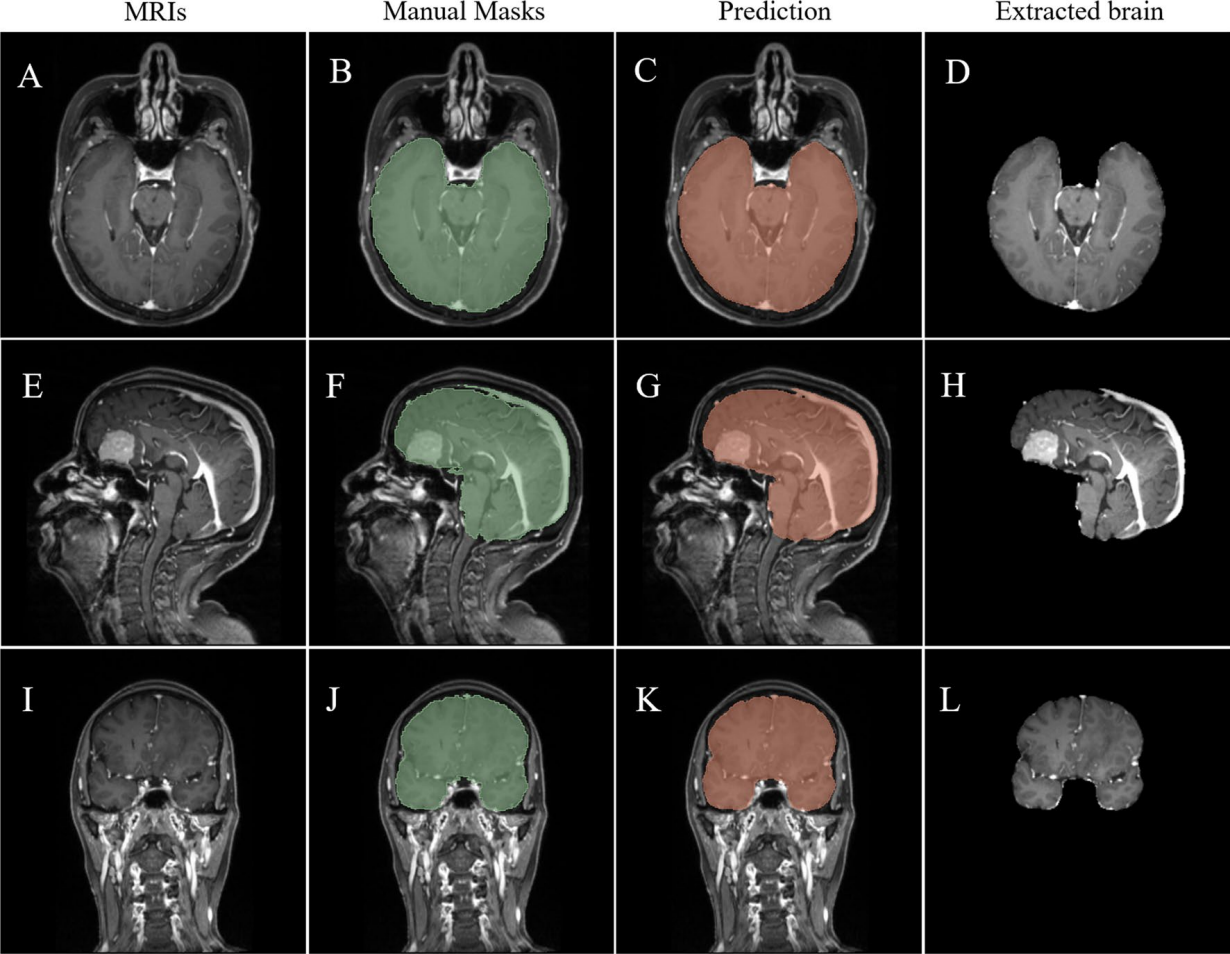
A brain extraction example for skull base meningioma from internal test. A–D Axial views. E–H Sagittal views. I–L Coronal views. Segmentation performance of this case is DSC of 0.991, HD of 5.196 mm. MRIs, magnetic resonance imagings
